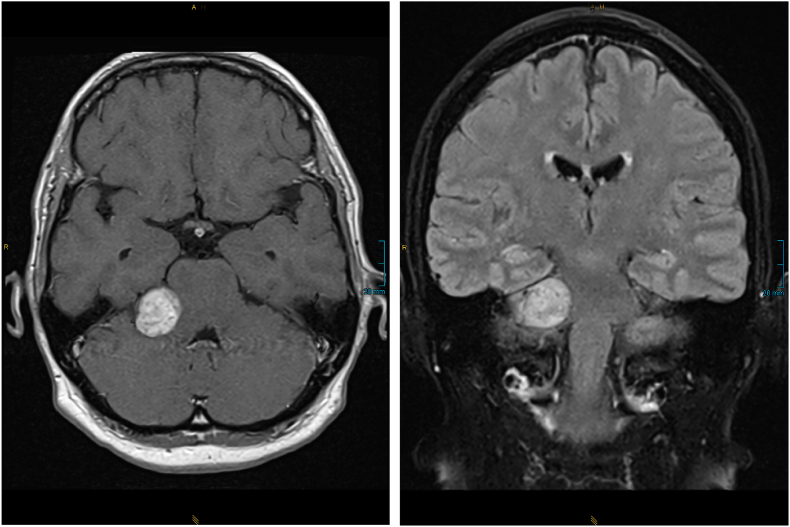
Preoperative MRI: axial T1weighted image post contrast and coronal FLAIR weighted image showing the tumor in the cerebellopontine angle.
